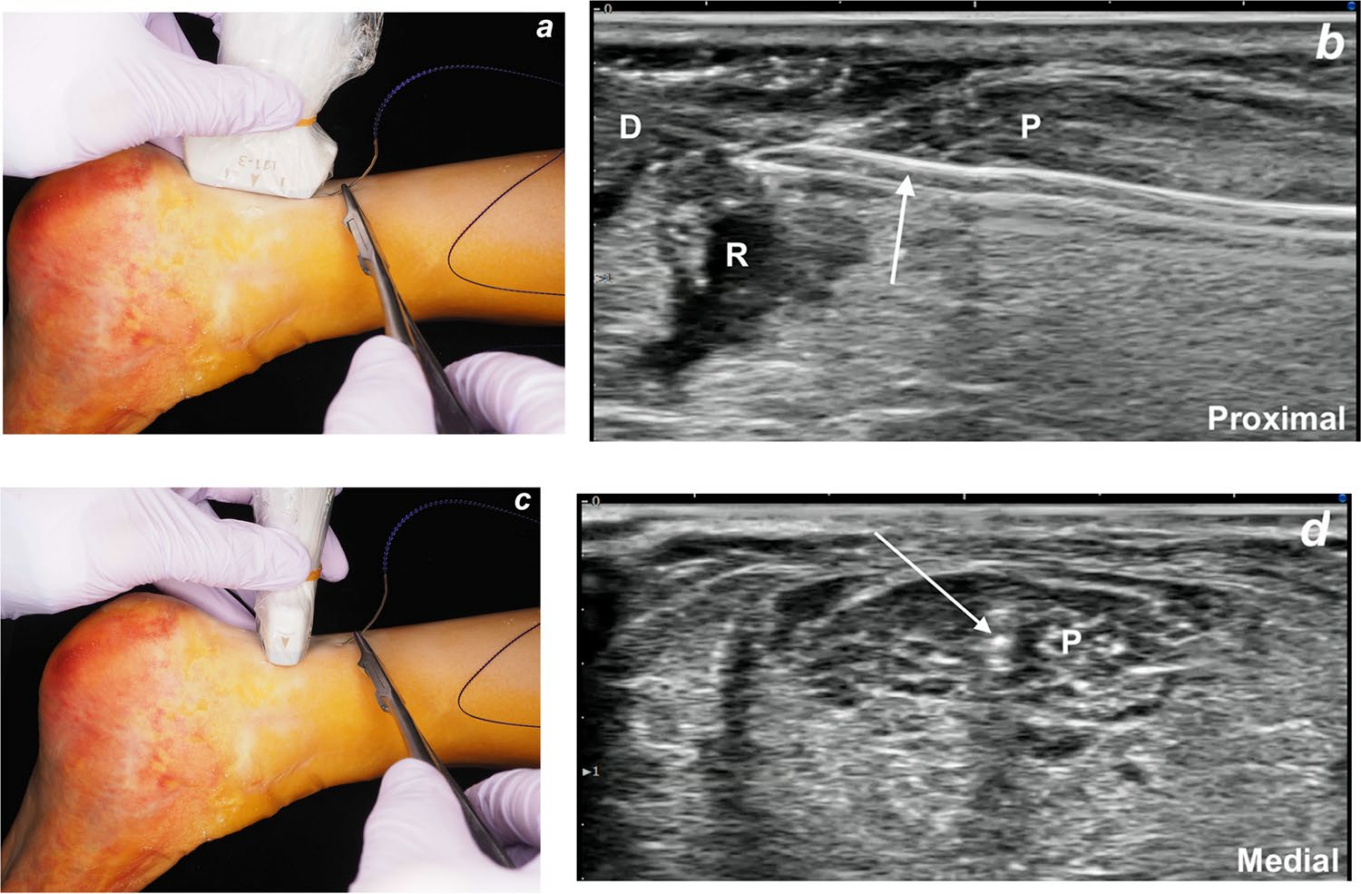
a, b, c, d Probe positioning in the longitudinal axis (a) with the corresponding ultrasound image showing intratendinous needle advancement (b), and probe positioning in the short axis (c) with the corresponding short-axis ultrasound image (d). Arrow: needle, D: distal end of the tendon, P: proximal end of the tendon, R: rupture site
